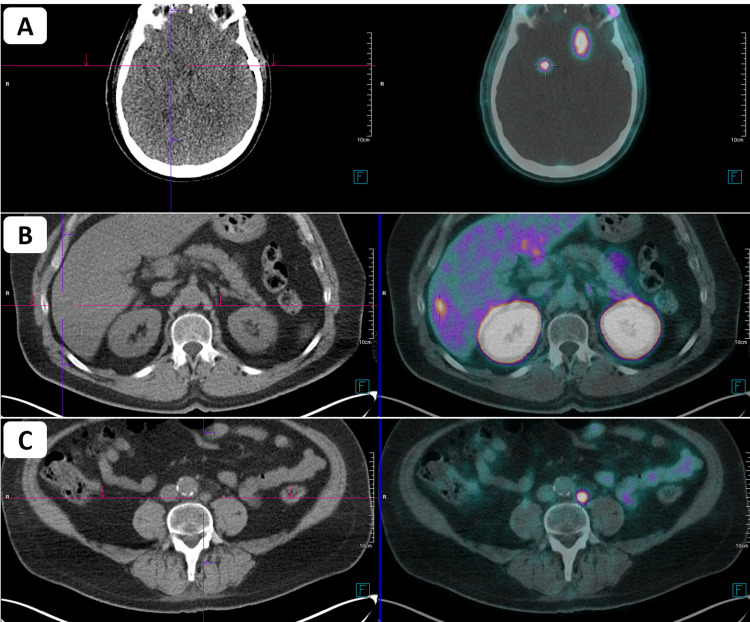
PET-PSMA images demonstrating disease progression after excision of the fronto-basal lesion at the brain, with bilateral cerebral metastases (A), suspicion of liver metastases (B) and lumbo-aortic lymph node metastases (C). PET-PSMA: positron emission tomography-prostate-specific membrane antigen
